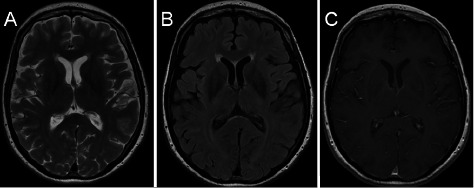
Axial Brain MRI sequences after treatment. A: T2 weighted image, B: FLAIR, C: post contrast T1 image showing resolution of previously noted lesions.
